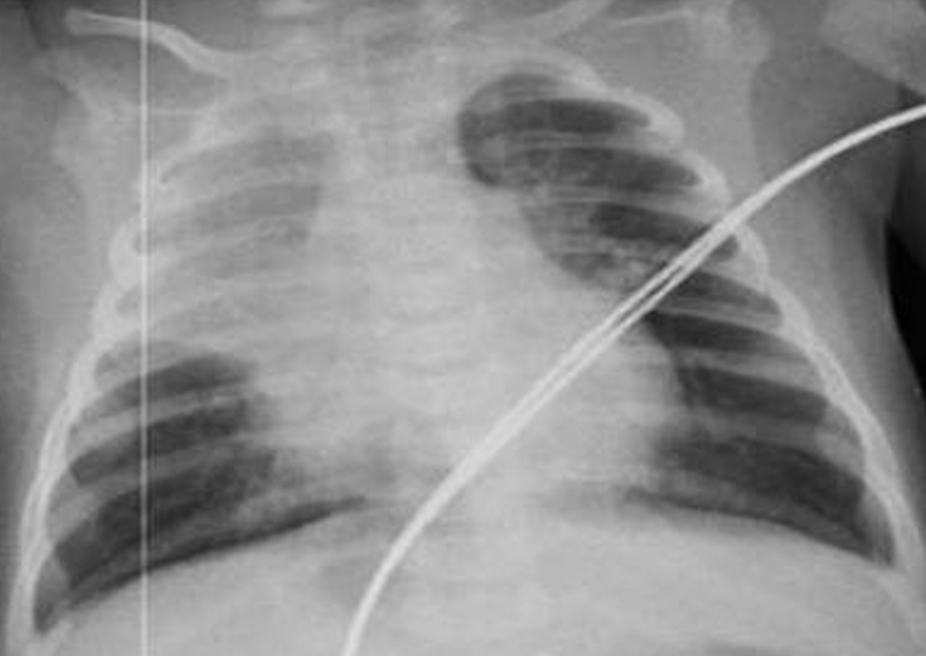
Chest X-ray of case 3.
